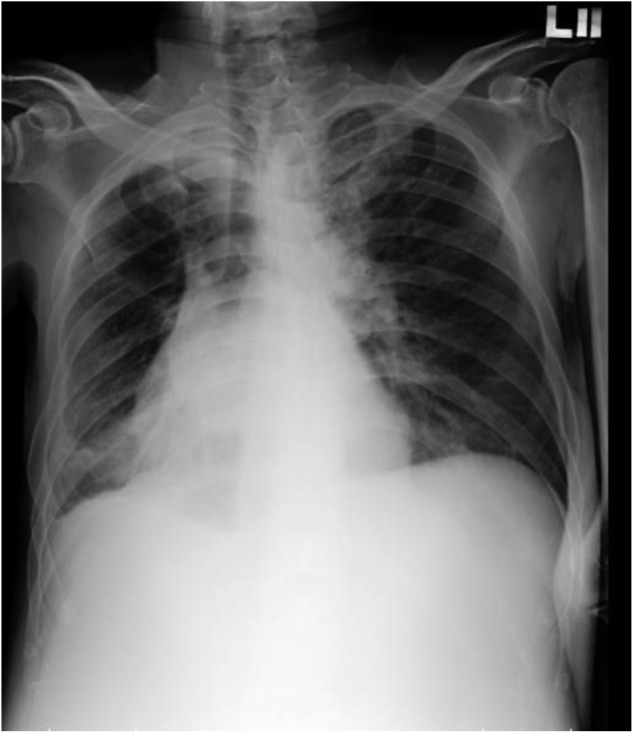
Chest X-ray revealed a lower lobe infiltrate and mild blunting of C-P angle in right side.
